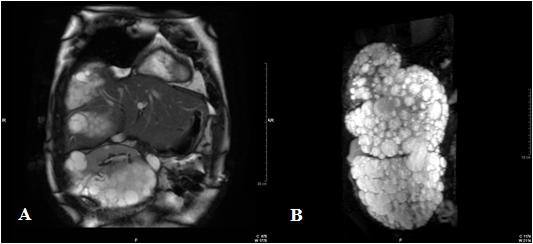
(A) Axial steady state sequence shows the cyst adherent to the right hepatic lobe and to the right kidney which is displaced into the pelvis, (B) Three-dimensional T2-weighted sequence shows the giant multiloculated liver cyst with its exophytic growth.
